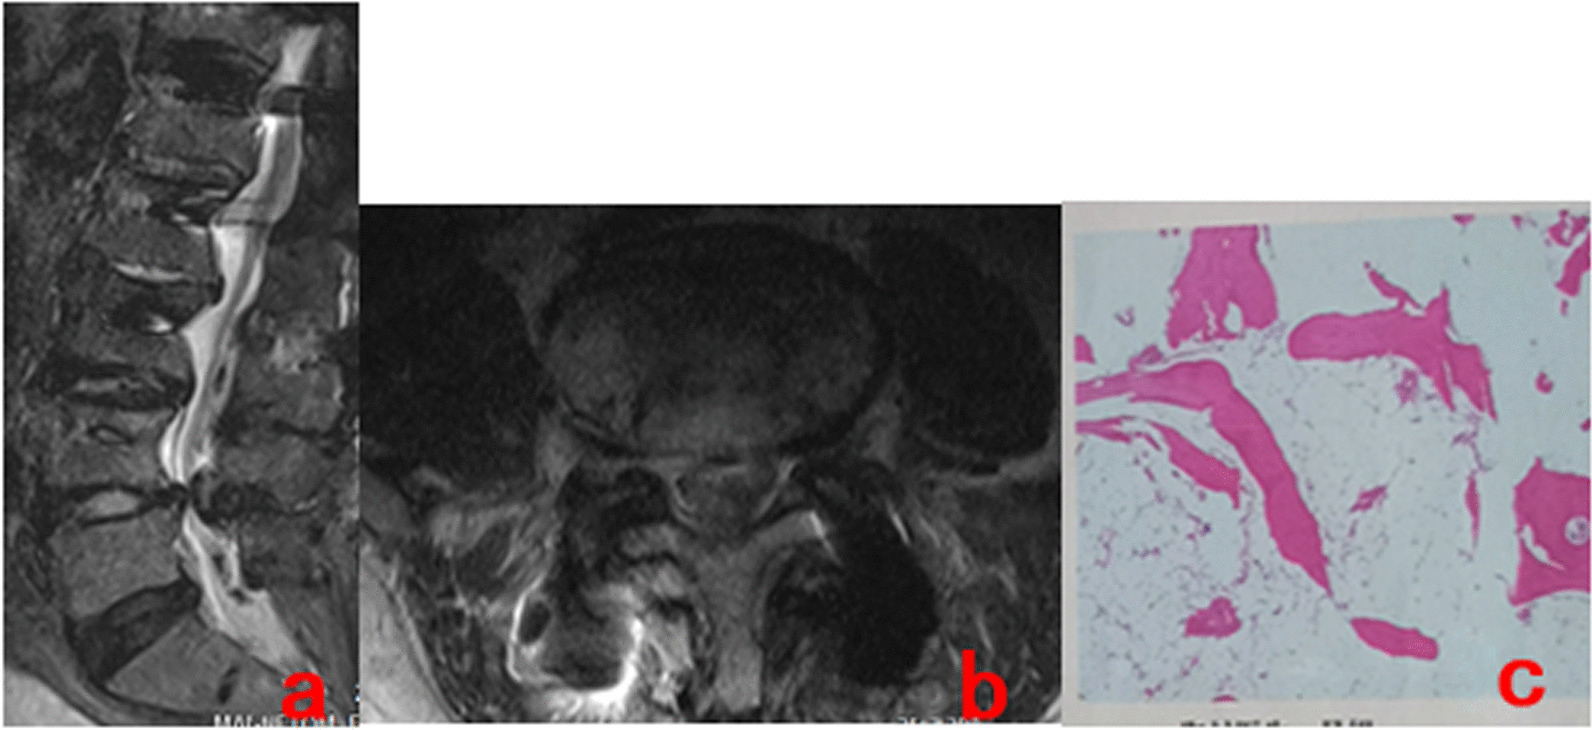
a, b MRI,3 days after operation, showed that the mass in the L4/5 spinal canal was completely removed, and edema signals were seen in the operation area. c Postoperative pathological examination showed fibrous tissue hyperplasia with vitreous degeneration and local mucus degeneration
